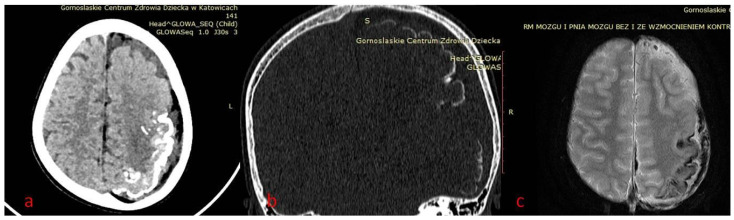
(a–c) Patient 1. Brain CT—left parietal and temporal gyral calcifications. (a,b) CT in axial brain window and coronal reformatted bone window; (c) MRI SWI sensitive to calcium/blood metabolites.
